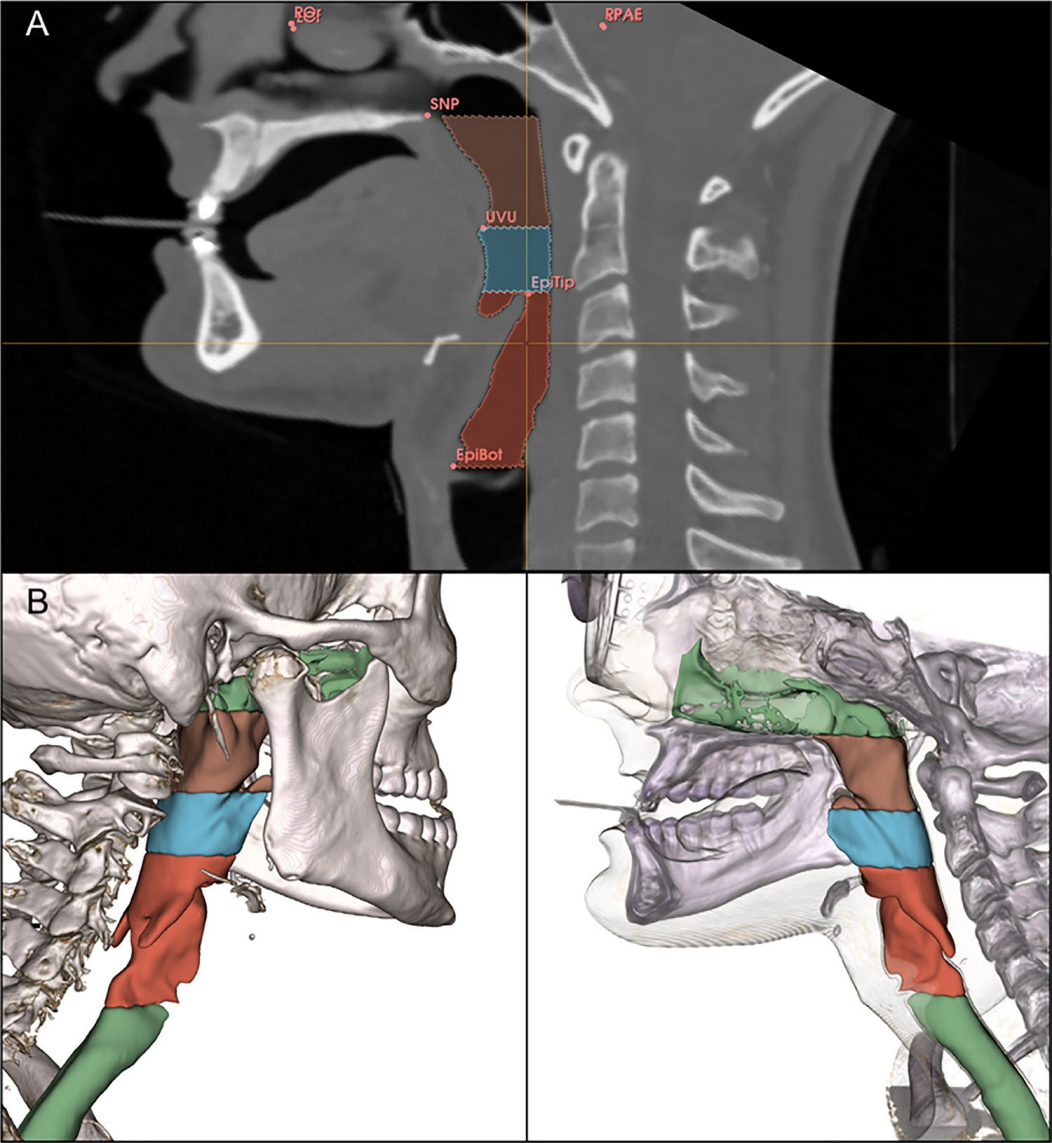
(A) Following anatomical landmarks represented the boundaries of the pharyngeal airway and were used for CT-scan alignment and measurements: SNP = *posterior nasal spine*; RPAE = *right porus acusticus externus*; UVU = *uvula tip*; EpiTip = *epiglottal tip*; EpiBot = *epiglottal bottom*. (B) Three-dimensional rendering of the pharyngeal airway without (left) and with (right) soft tissue contour
